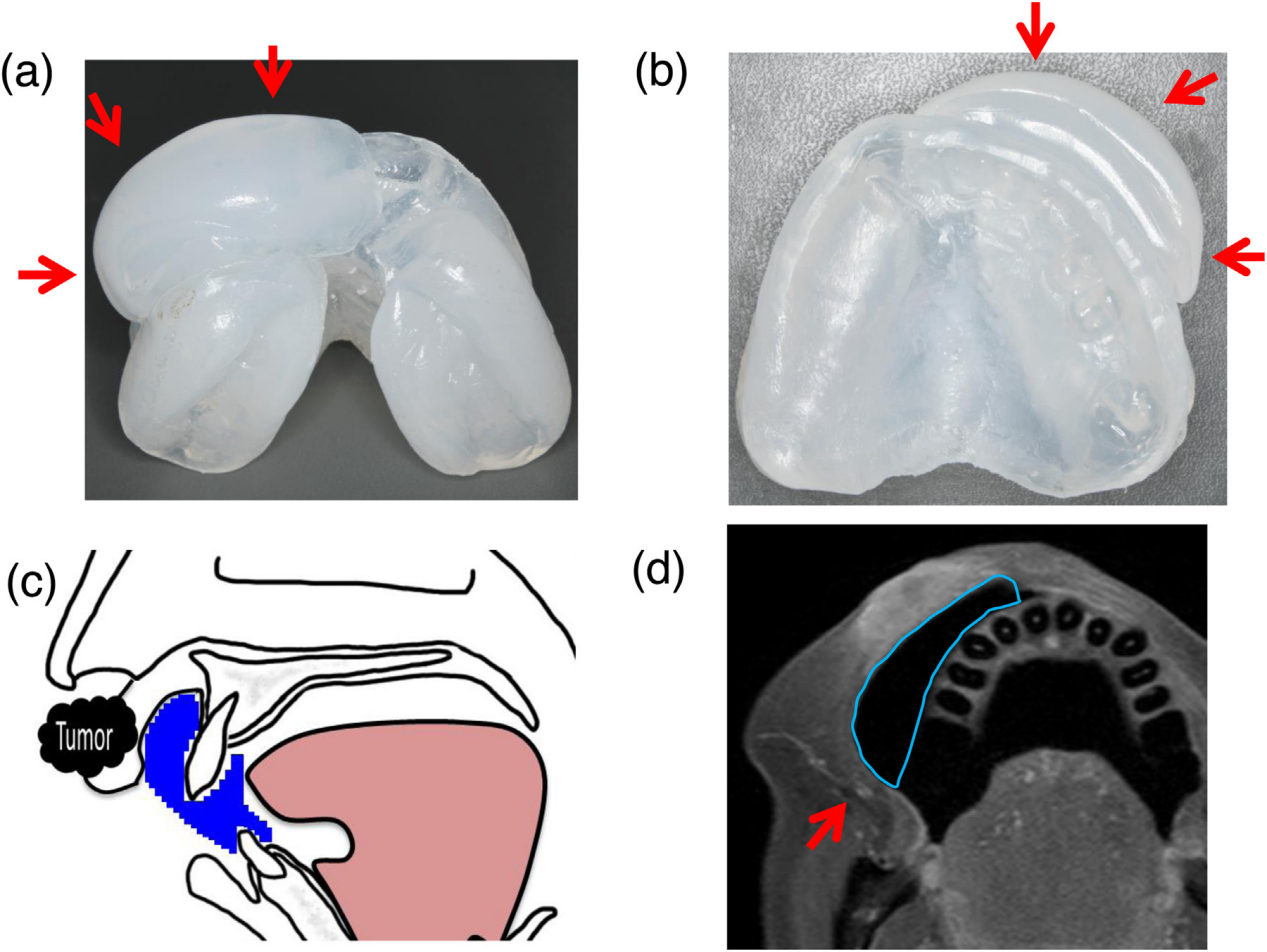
Custom-made lip bumper to protect the maxilla from irradiation. (A) Mouthpiece with lip bumper from the lingual side (arrow). (B) Mouthpiece with lip bumper from the palatal side (arrow). (C) Bumped lip position with custom-made mouthpiece. (D) T1-weighted contrast-enhanced axial magnetic resonance image of lip tumor with lip bumper.
